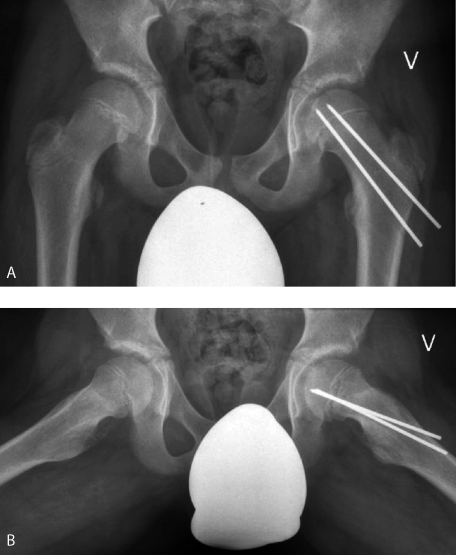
Postoperative radiographs of a 14-year-old boy after percutaneous pinning of SCFE. A. supine AP view. B. Frog-leg view.
